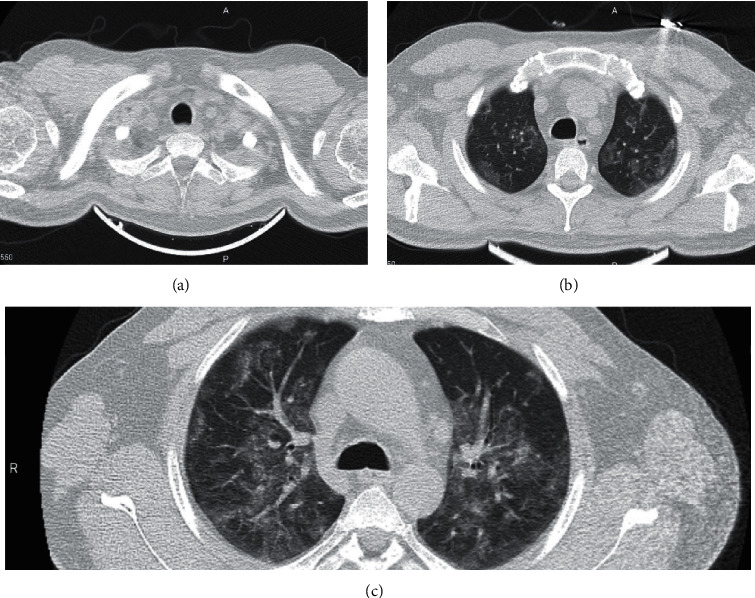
Axial images (a–c) of pulmonary computed tomography in the parenchymal window from the thoracic inlet and upper chest 2 months after the acute coronavirus infection. Complete resolution of the pneumomediastinum, pneumothorax, and soft tissue edema is evident. The persistence of the parenchymal opacities is predictable in all types of pneumonia including COVID-19-related ones.
